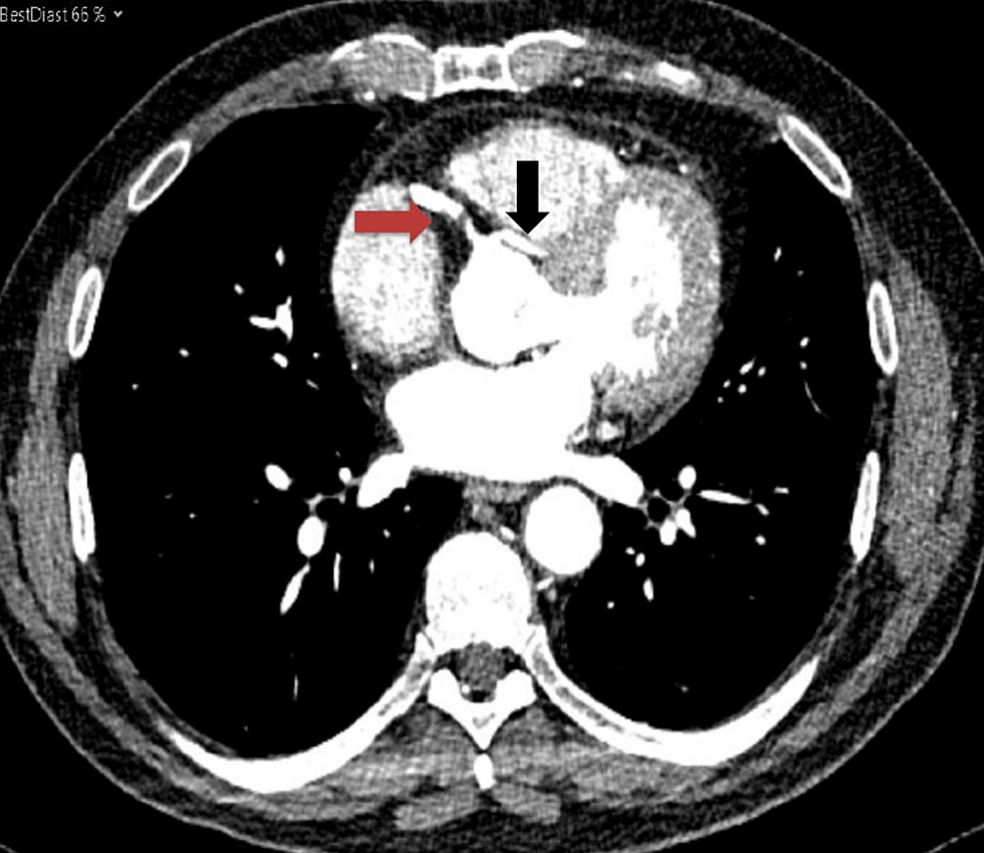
CT coronary angiogram showing the RCA (red arrow) and the anomalous origin of the left coronary artery (black arrow) CT: computed tomography; RCA: right coronary artery
